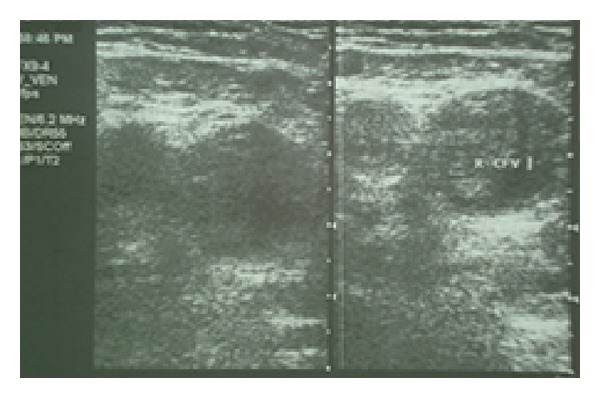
Triplexultrasound in patient B showing the thrombosis of the right common femoral vein (CFV). The vein is uncompressible.
